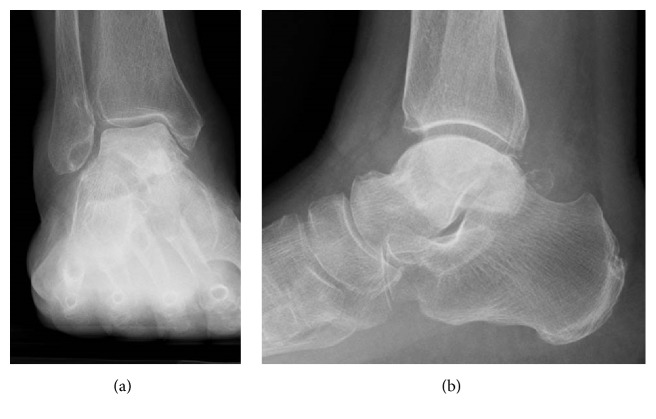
Preoperative radiographs showing sclerotic change of the talar body in anteroposterior (a) and lateral (b) views.
